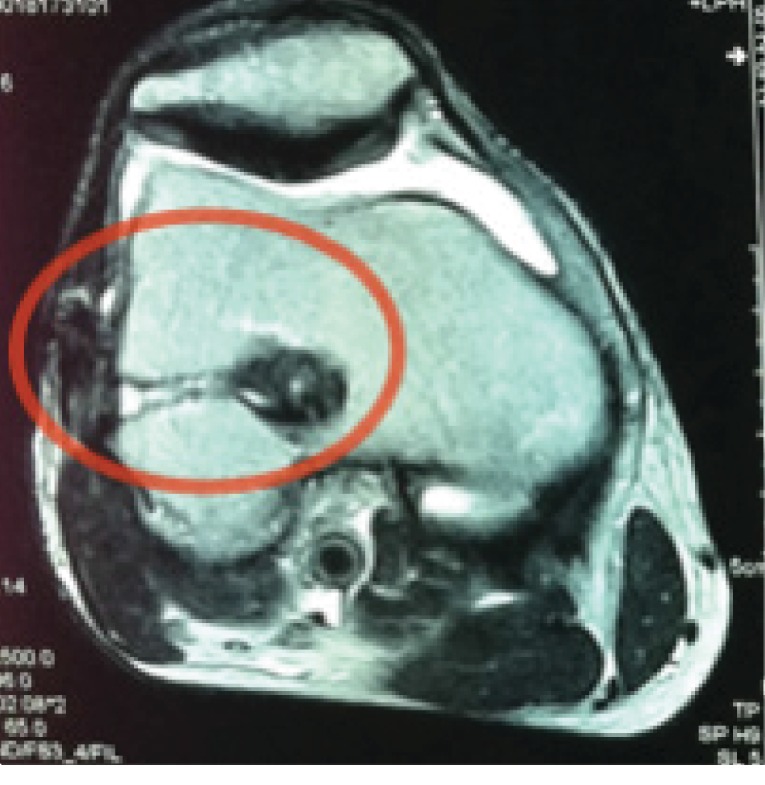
Magnetic resonance image (MRI) showing the fistula, marked with a red circle, that connects the ACL graft femoral tunnel to the cortical bone. The tunnel is filled with synovial fluid which comes from inside the joint. This image also shows the soft tissue disruption in the lateral aspect of the femoral condyle.
